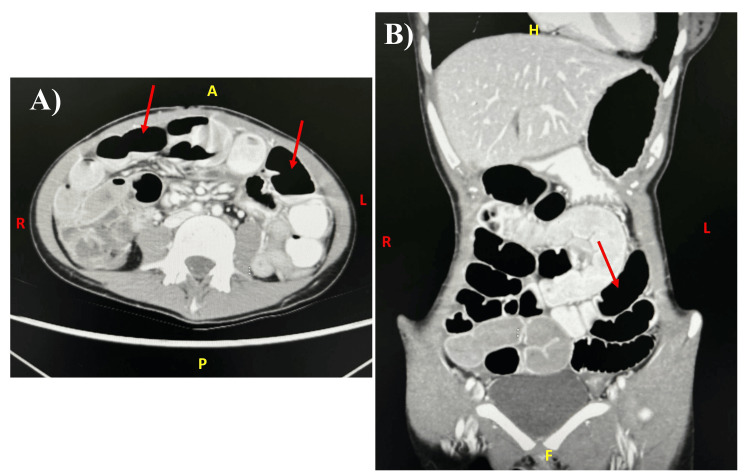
The abdominal computed tomography (CT) of the studied case CT showed a diffuse dilatation of the small bowel loops, which affected most of the jejunum and ileum (red arrows). A: axial view; B: coronal view
